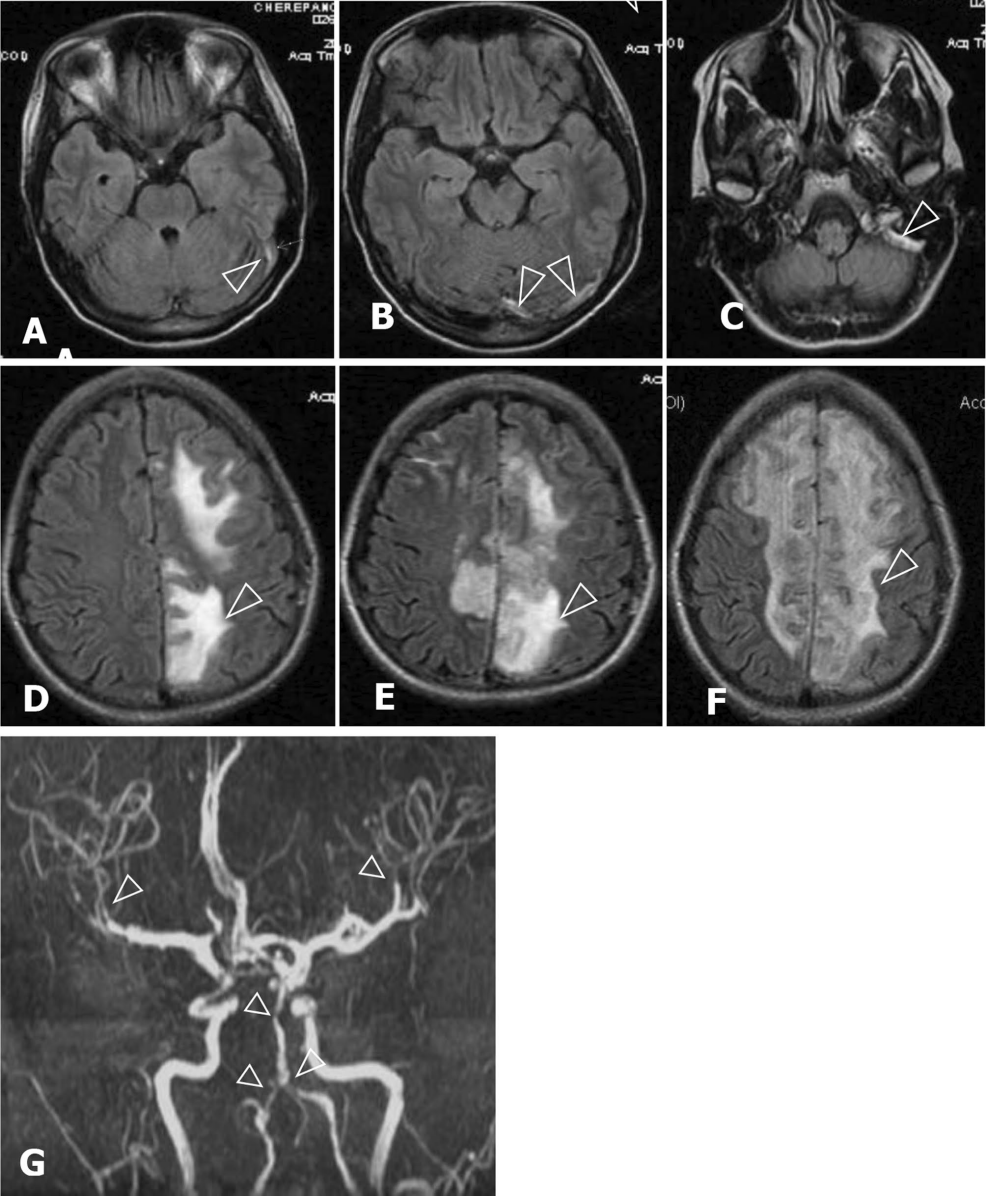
Cerebral venous sinus thrombosis (left transverse and sigmoid sinuses) in case 1. A–C FLAIR: areas of the increased signal intensity at the sinus location at 10. day of encephalopathy. D, E FLAIR: progression of focal lesions within 1.5 months (D 10. day, E 17. day, F 50. day of encephalopathy) leading to diffuse gliosis. G 3D-time of flight (TOF) MR angiography: vascular narrowing and uneven shapes of basilar and both vertebral arteries suggesting segmental vasospasm at the 10 day
